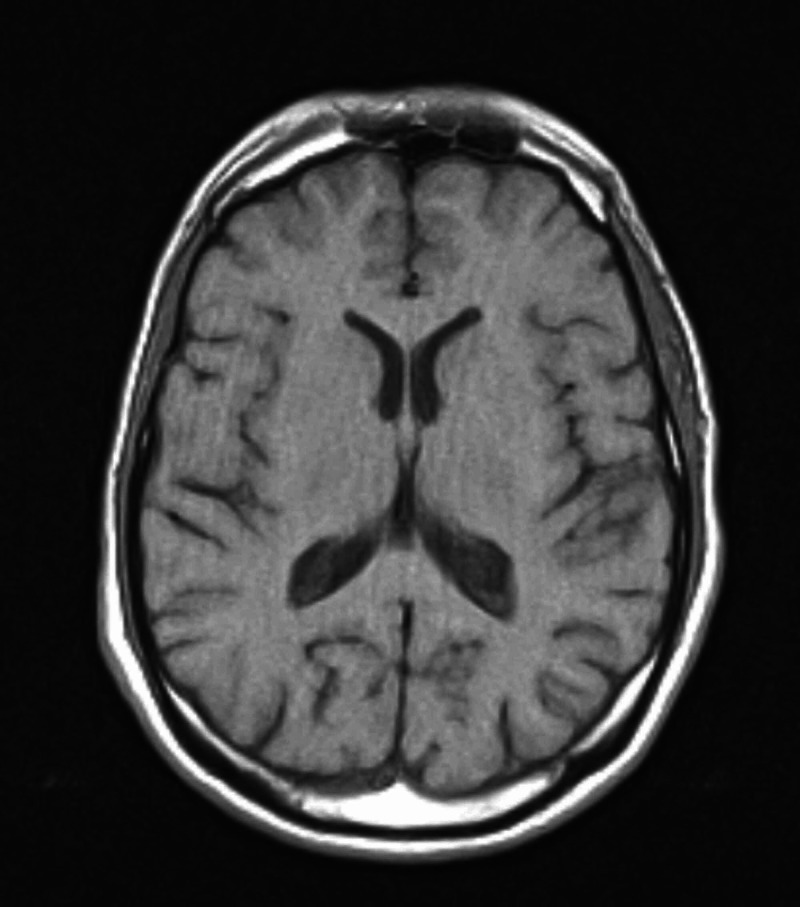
A transverse plane MRI of the patient’s brain.
